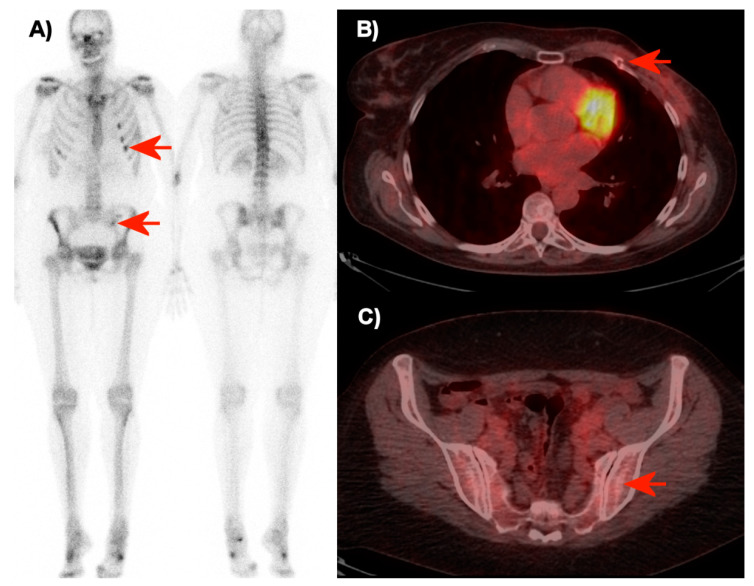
Bone scintigraphy (A) and transaxial FDG-PET/CT images over the thorax (B) and pelvis (C) of a 50-year-old woman without metastases (seven months of follow-up). The raters disagreed in the interpretation of the bone scintigraphy (A). Rater 2 interpreted these with point assessment 2 (probably metastases), while rater 3 interpreted the small focus in the left side of the pelvis and the successive foci in ribs (red arrows) with point assessment 1 (probably no metastases). On FDG-PET/CT (B + C), the two raters agreed about point assessment 0 (no metastasis). The focal FDG-uptake in the ribs was interpreted as sequelae of non-malignant traumatic fractures, and no focal FDG-uptake or structural pathology was observed in the left part of the pelvis on FDG-PET/CT (red arrows).
